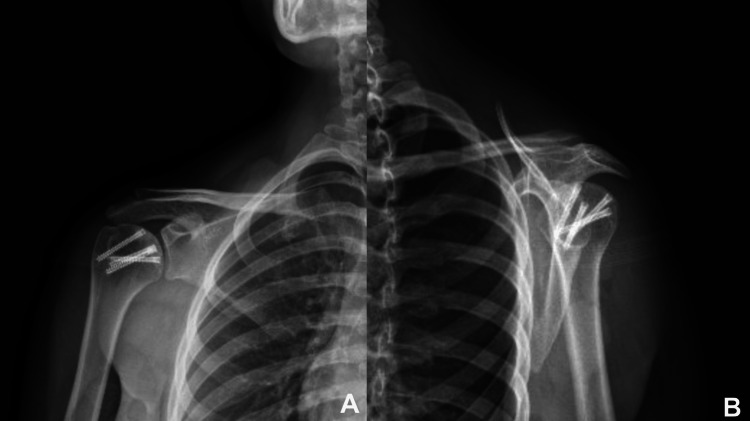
Follow up anteroposterior (A) and axial (B) radiographs No radiologic signs of arthrosis are evident.
